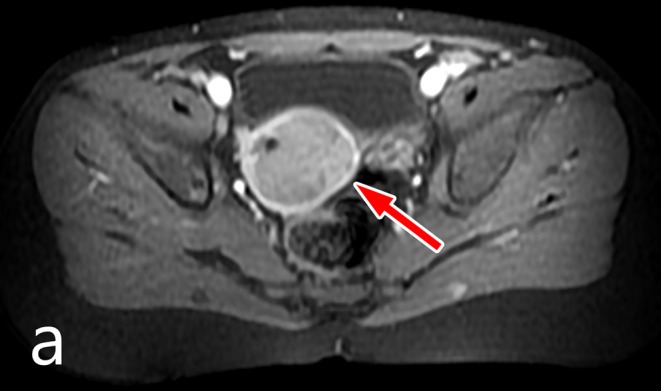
MRI image of the uterine lesion.
